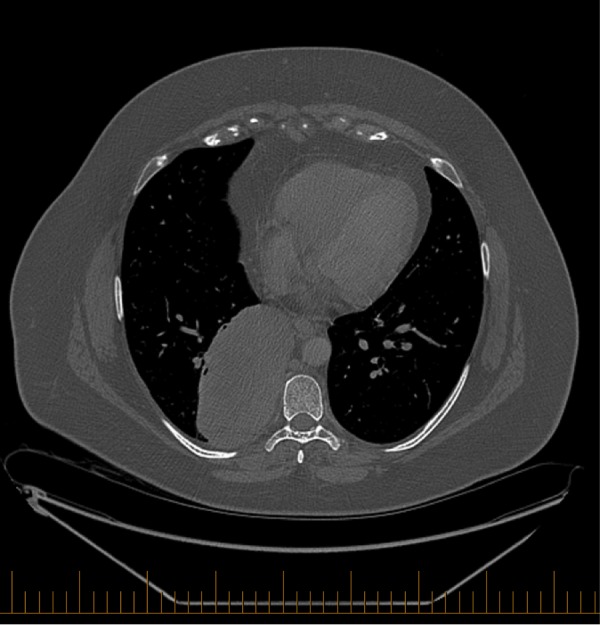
Axial CT scan of the chest showing a 10-cm paravertebral, subpleural thoracic mass.
